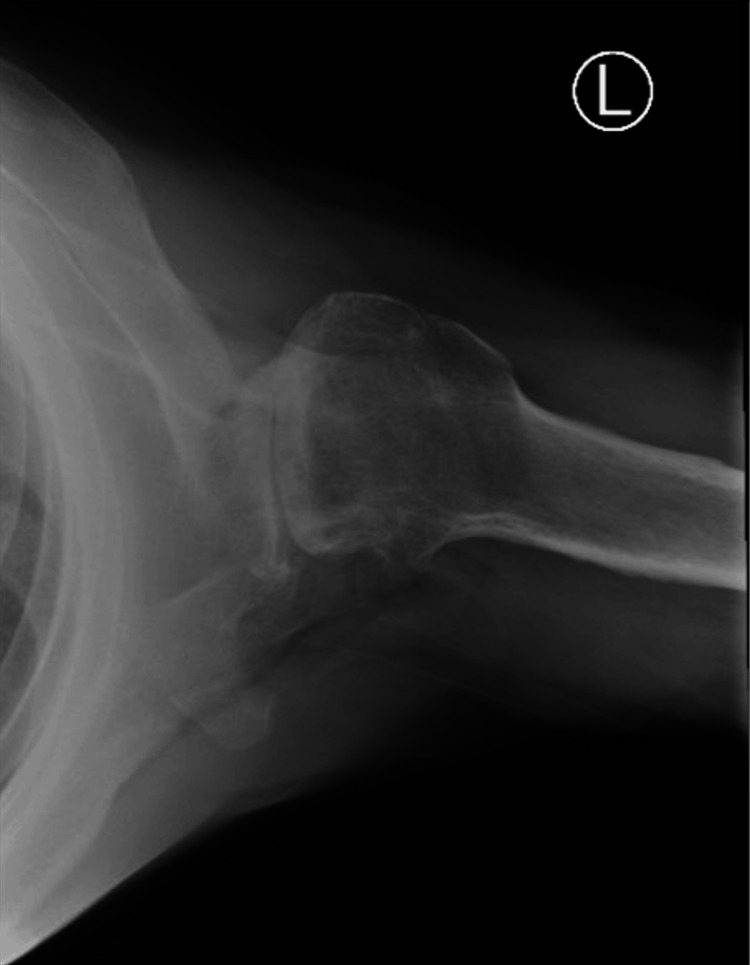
Preoperative X-rays of the left shoulder The image depicts an axillary view of the left shoulder
